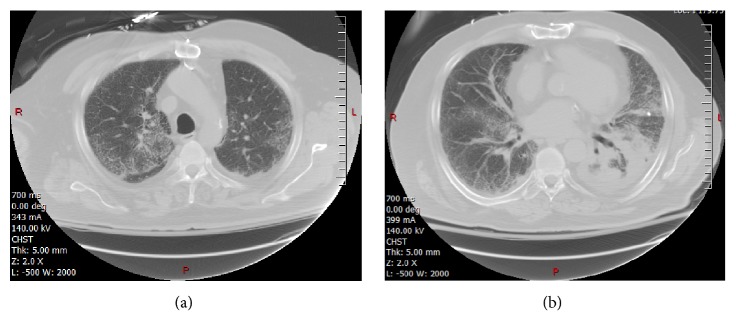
CT images above and at the plane of SBRT treatment showing lung infiltrates and dense consolidation in the left lower lung ((a) and (b) images respectively).
